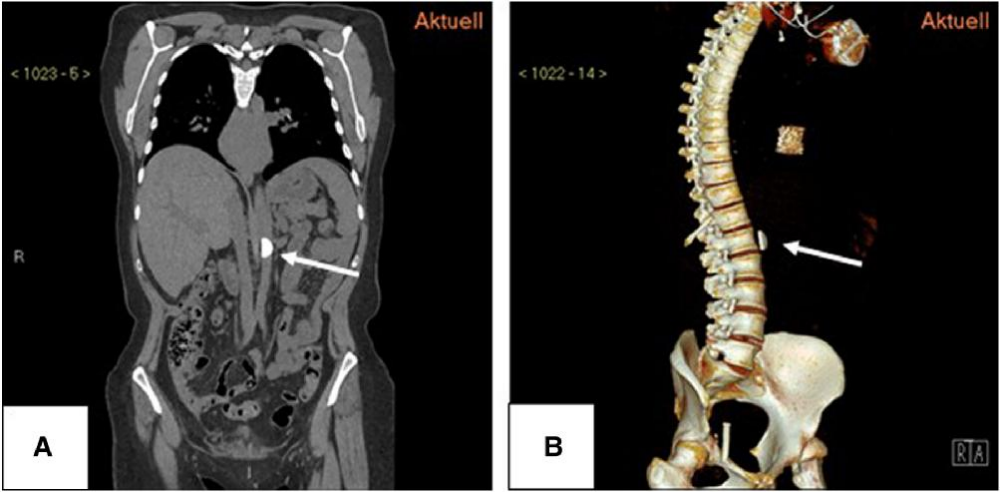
CT scan of the chest and abdomen in frontal plane (*A*) and oblique (*B*) showing the tilt (white arrow) in abdominal aorta.
